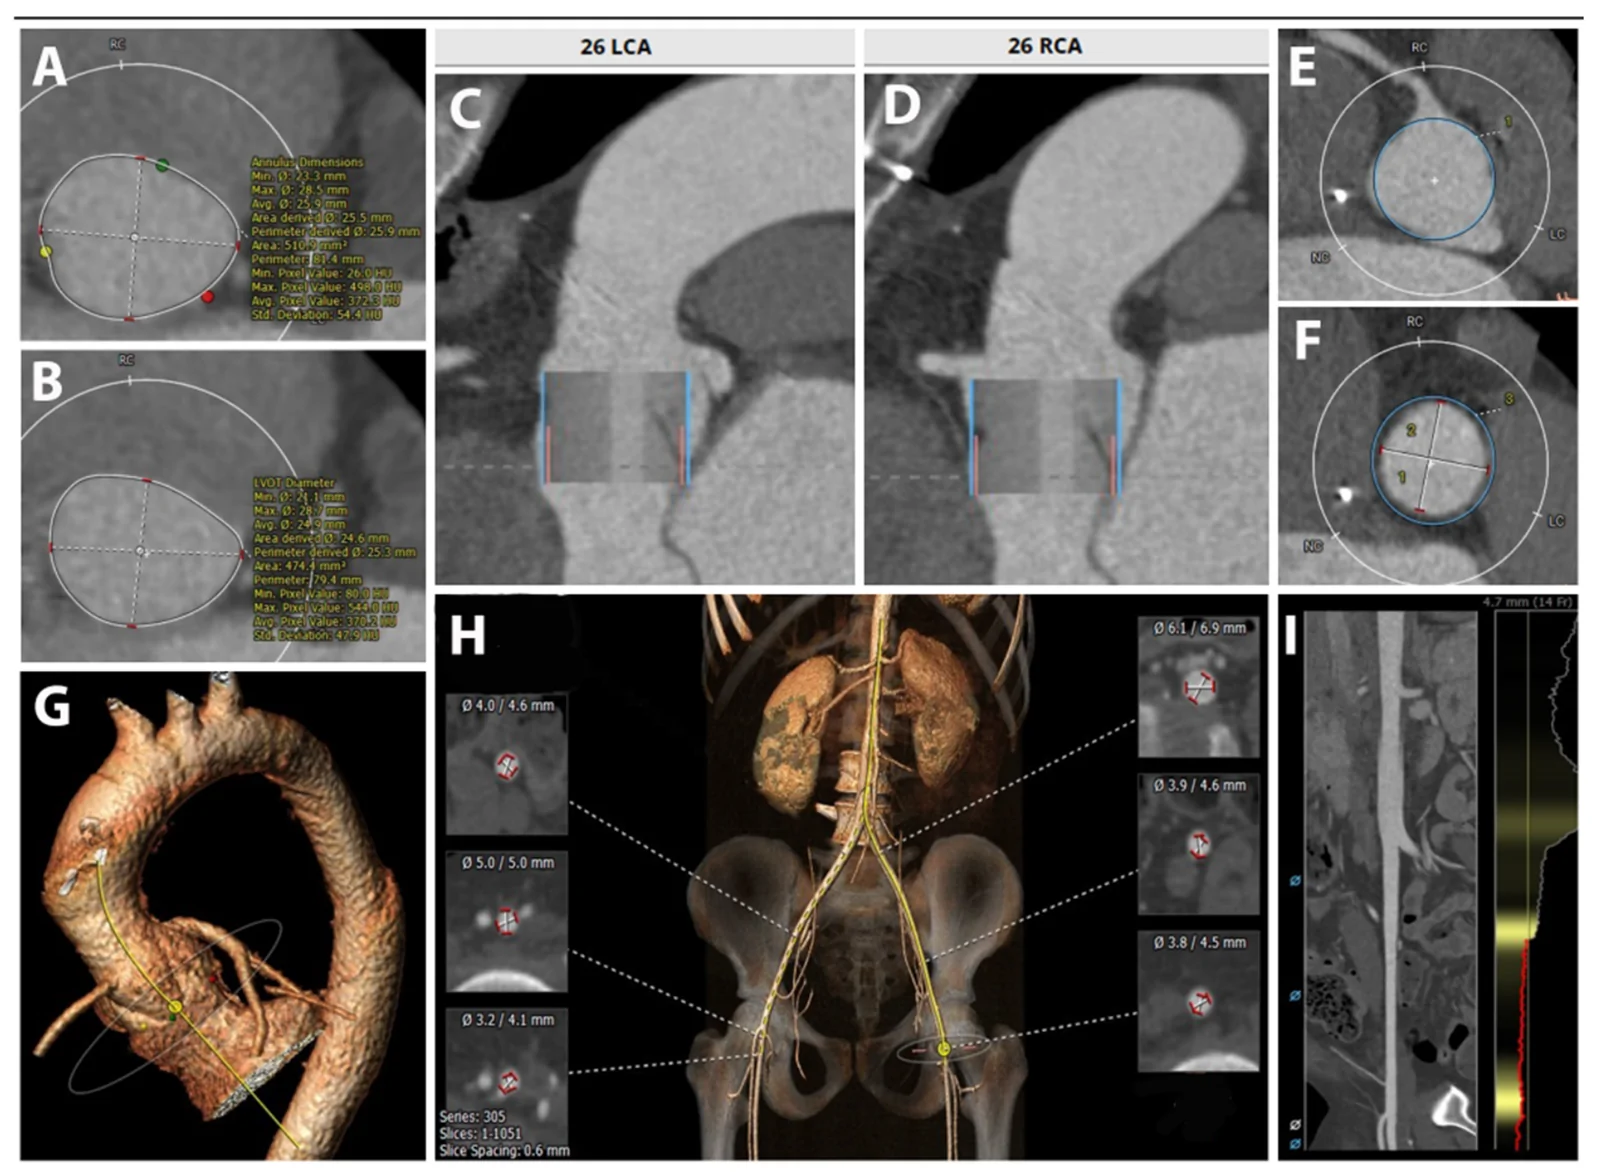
Multidetector computed tomography (MDCT) assessment for preprocedural TAVI planning. CT analysis included annular and LVOT sizing (A,B), coronary ostial height assessment (C,D), sinuses of Valsalva and sinotubular junction evaluation (E,F) in relation with valve projection; three-dimensional reconstruction of the aortic root for assessment of the optimal fluoroscopic implantation angle (G); assessment of iliofemoral access with vessel diameter measurements and curved multiplanar reconstruction confirming suitability for transfemoral TAVI (H,I).
